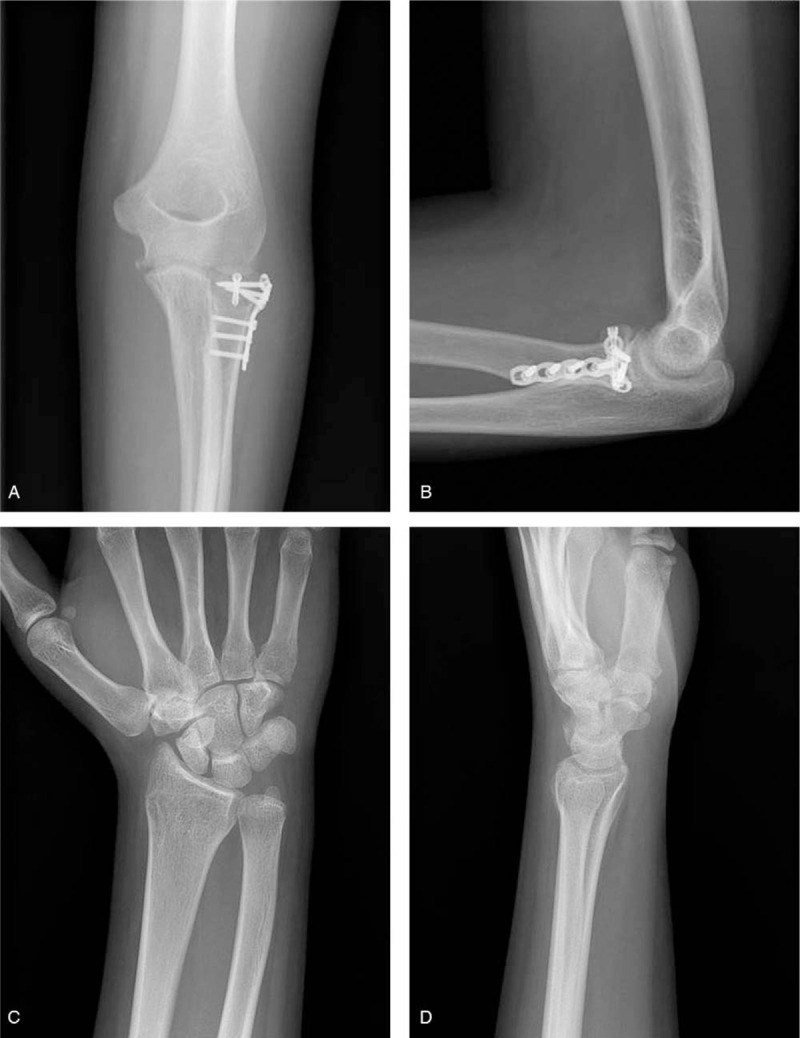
Postoperative plain radiographs of the elbow (A, B) of case 2 show that open reduction and internal fixation was performed for radial head fracture. Distal radius fracture was treated conservatively and the final plain radiographs show good bony union (C, D).
